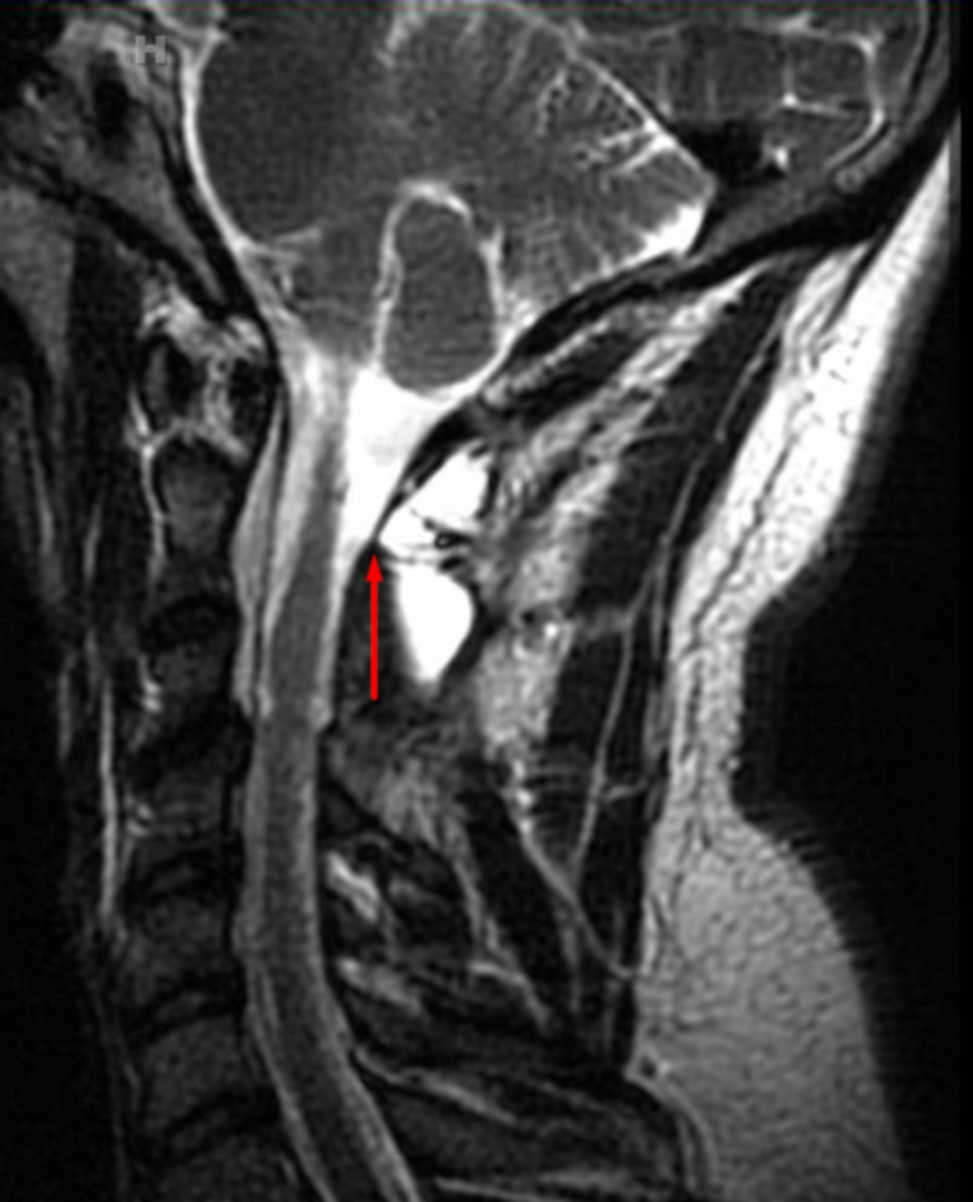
Cervical spine sagittal MRI without contrast showing a near-complete resolution of the pseudomeningocele after dural repair with BMP MRI (Magnetic Resonance Imaging), BMP (Bone Morphogenetic Protein)
